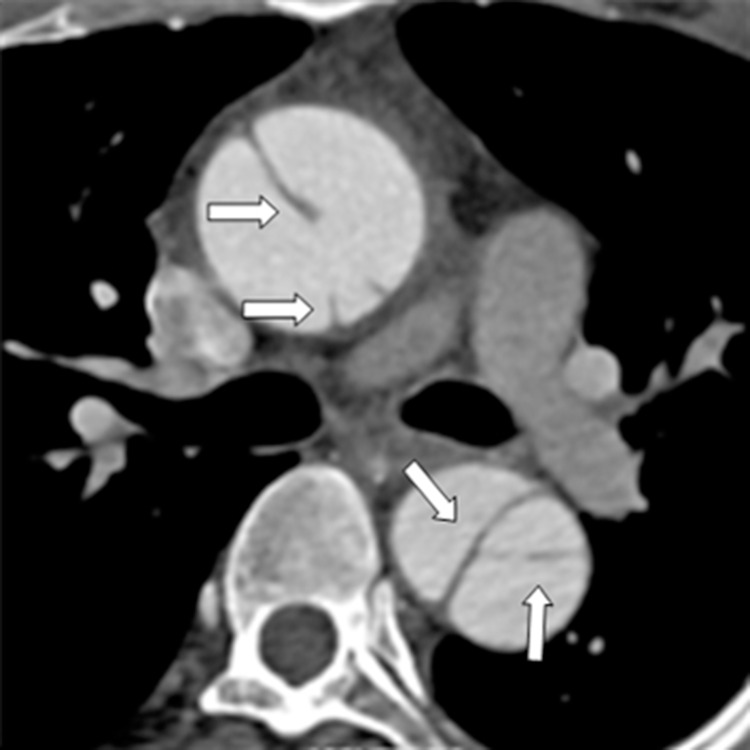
Multiple-line type of the intimal flap on an axial CT image.
